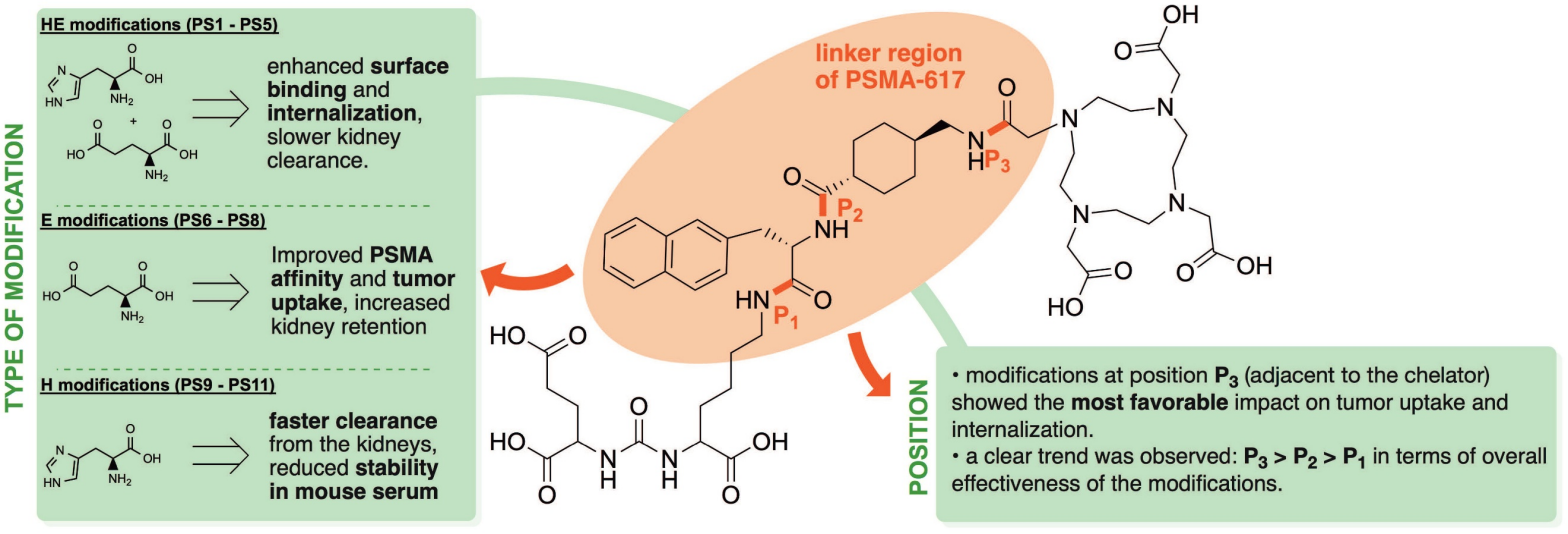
Summary of structure-activity relationships derived from experimentally observed trends in *in vivo* and *in vitro* experiments for PSMA-617 analogues with various linker modifications.
